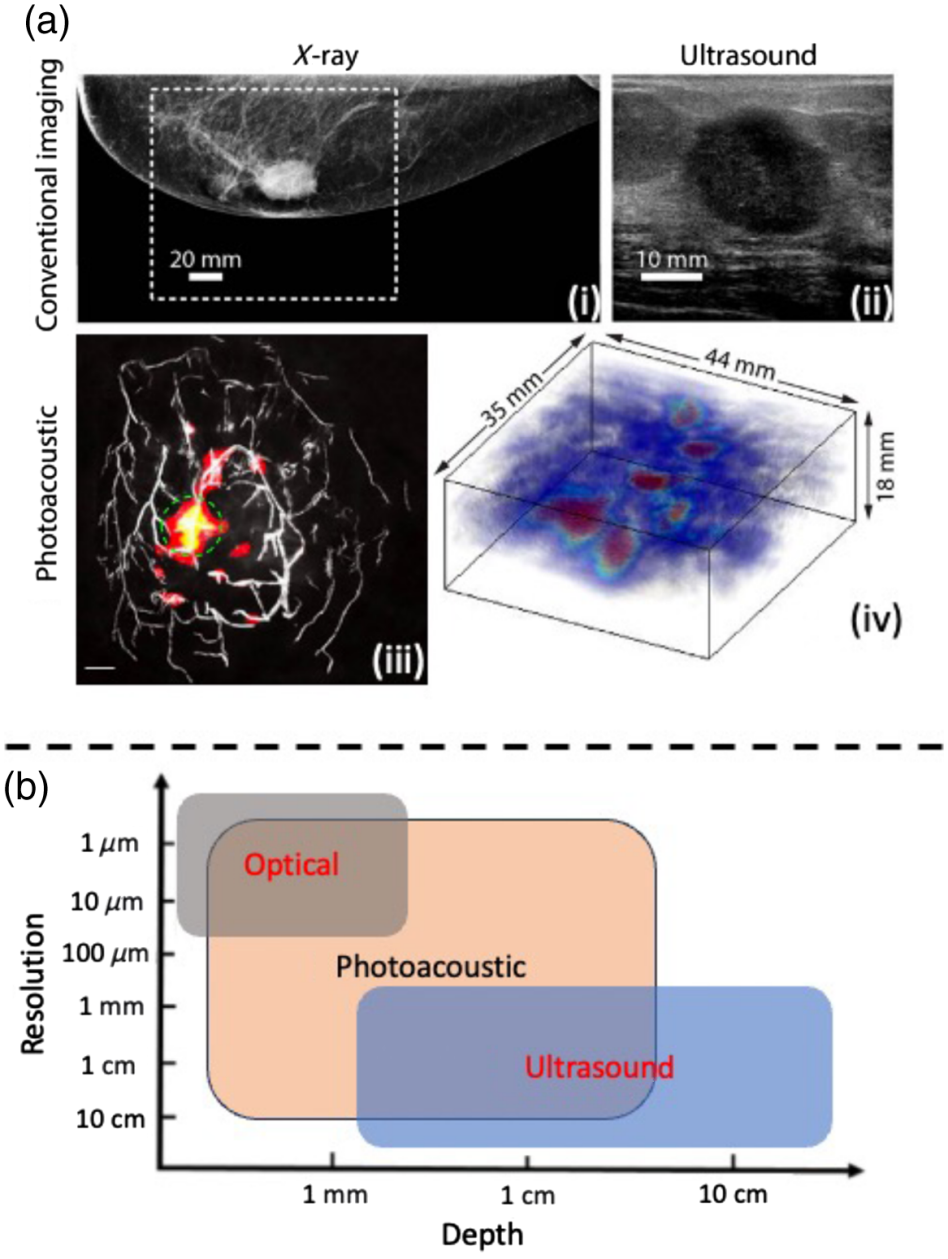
Comparison between X-ray, US, and PA imaging modalities. Reprinted with permission from Refs. 5–7. (a)(i) The X-ray image of the left breast displays a suspicious mass, with the white box indicating the field of view for the PA image. (a)(ii) The US image of the palpable mass confirms a highly suspicious mass. (a)(iii) The MAP of the PA volume depicts vessel density maps with tumors identified by a green circle. (a)(iv) A 3D volume rendering of the PA image exhibits a distinctive ring-like appearance. (b) Evaluation of resolution and depth characteristics across various imaging modalities, including US, optical, and PA imaging.
